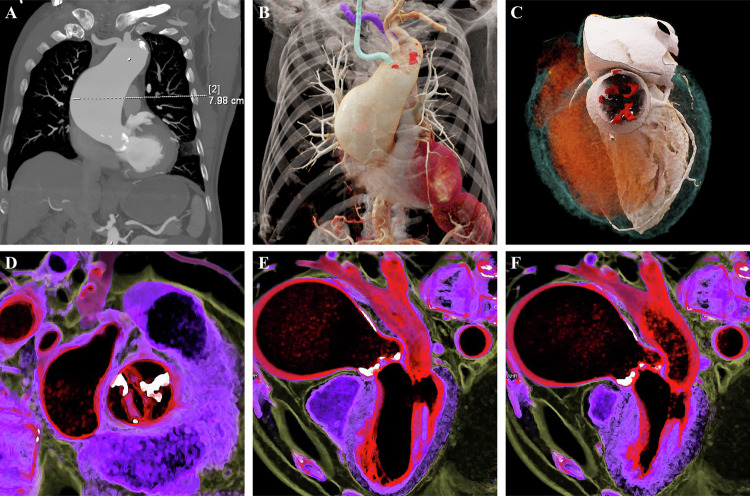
Chest CTA of a 78-year-old male with ascending aortic dilation and aortic valve calcification. A The MIP image shows a measurement indicating dilation of the ascending aorta to approximately 7.88 cm, with visible calcification of the aortic wall and valve. B The CR image intuitively visualizes the dilated morphology of the ascending aorta and mural calcified plaques (highlighted in red). It also clearly depicts anatomical variations, including an aberrant right subclavian artery (highlighted in purple), within the same field of view. C The CR image focuses on the aortic valve, stereoscopically displaying the distribution of calcified foci (red). D–F Dynamic cinematic rendering (4D-CR) of the aortic valve captures images at different phases of the cardiac cycle, dynamically demonstrating valve calcification and resultant motion restriction. Notably, during systole (D, F), valve opening is significantly restricted, providing an intuitive dynamic anatomical basis for valve replacement surgery
